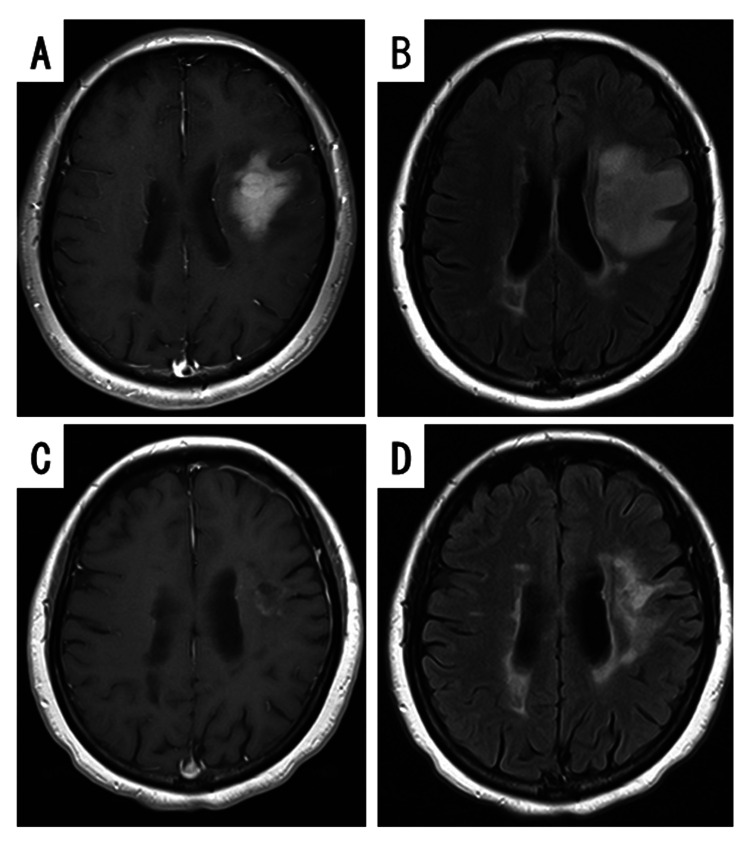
Gadolinium-enhanced (Gd+) and FLAIR MRI before the surgery and after chemotherapy Preoperative MRI displayed a homogeneous Gd+ lesion (A), surrounded by a high-signal area of edematous changes on FLAIR (B) in the left frontal lobe. After the biopsy and three courses of R-MPV therapy, the lesion in the left frontal lobe had almost completely disappeared, although it had a slight contrast (C), and the high-signal area on FLAIR was also significantly reduced (D). FLAIR: fluid-attenuated inversion recovery; R-MPV: rituximab, methotrexate, procarbazine, and vincristine
